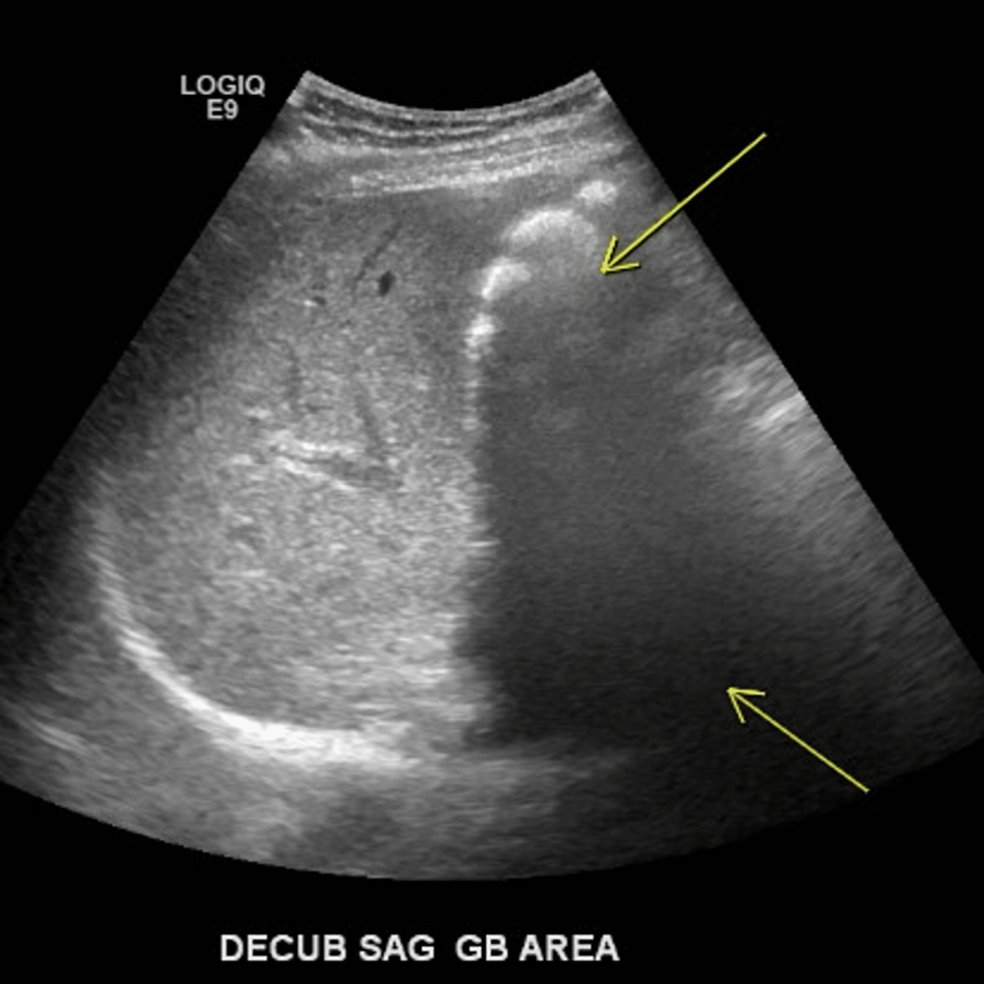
An ultrasound of the right upper quadrant demonstrates an absent gallbladder and stones. Shown is a sagittal decubitus view of the gallbladder area. There is no evidence of a localized gallbladder; however, there is shadowing, as indicated by the arrows, which is consistent with gallstones.
